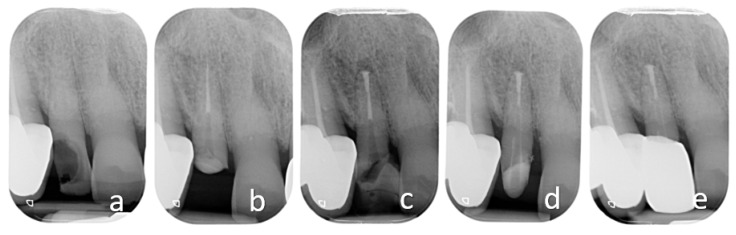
Surgical extrusion of 1.2 tooth with insufficient ferrule effect. Preoperative radiograph (a). Primary endodontic treatment (b). Reimplantation after retrograde endodontic treatment and splint (c). Tooth preparation and prosthetic rehabilitation after healing process (d,e).
